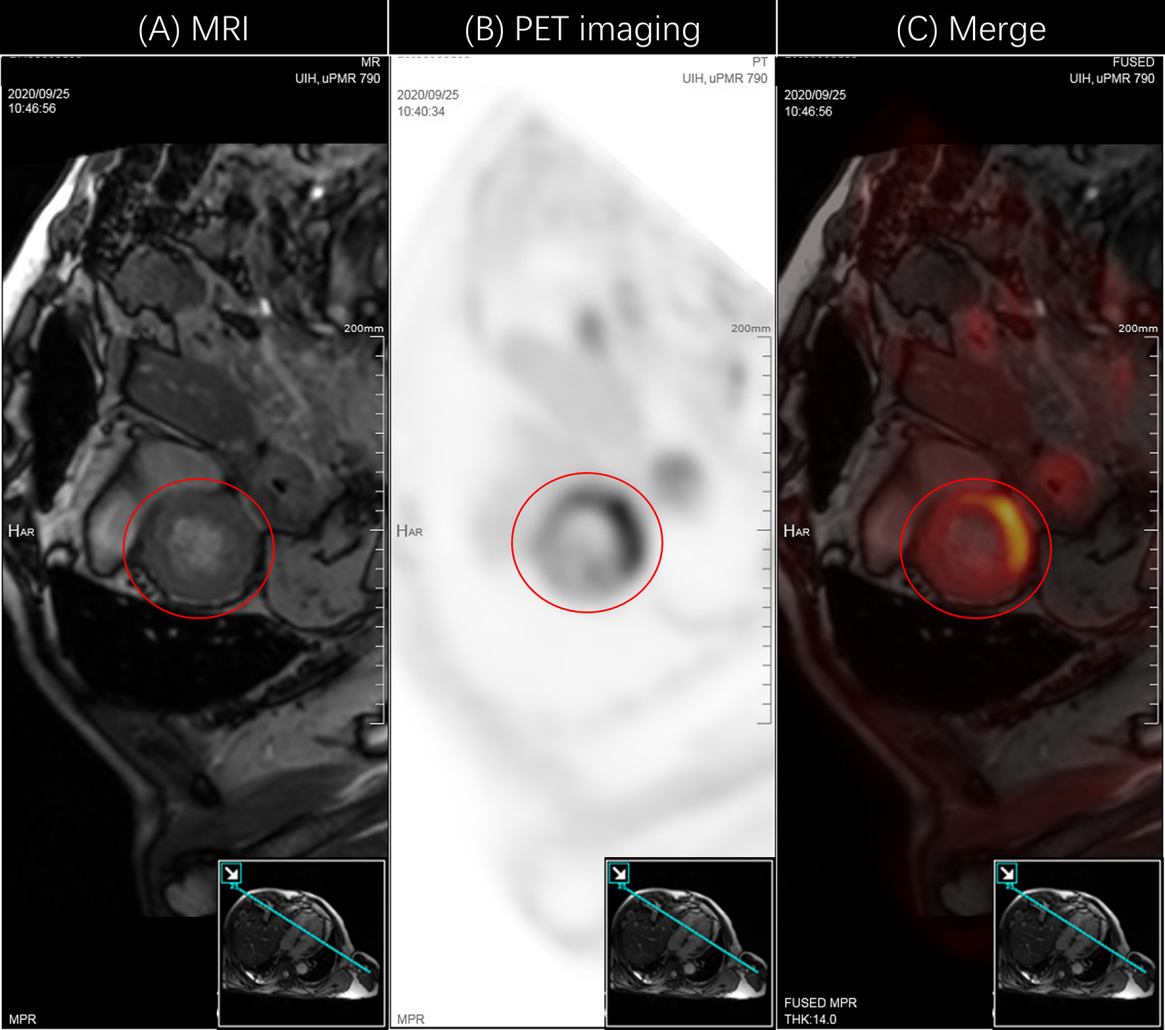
PET-CMR images showed myocardial inflammatory damage. (A) MR image indicated the physiological tissue structure of the heart. The red circle represented the left ventricle. The figure in the lower right corner was the image cut surface. (B) PET image indicated the uptake of the contrast media ^18^F-FDG. The red circle represented the increased intake. (C) Fusion image of PET and MR, which visually displayed myocardial inflammatory damage in the left ventricle. PET, positron emission computed tomography; ^18^F-FDG, ^18^F-flurodeoxyglucose.
